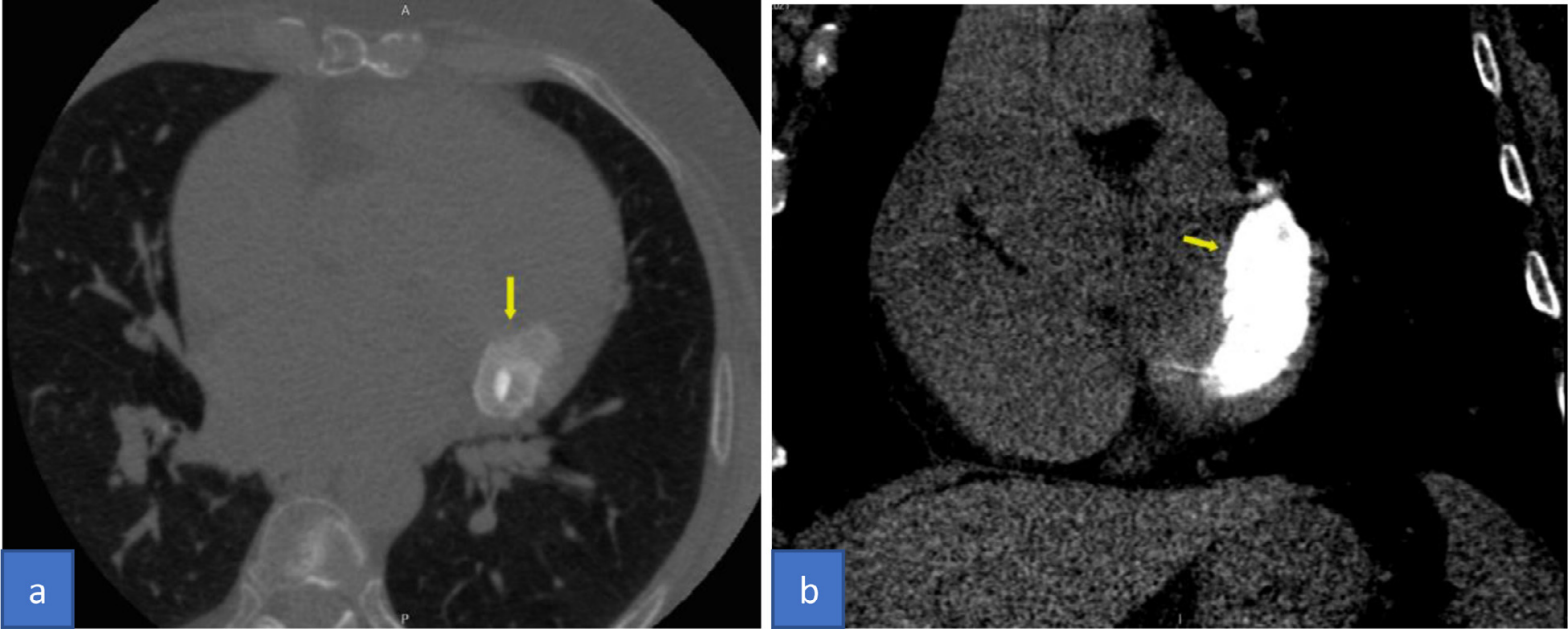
Computed tomography (CT) chest without contrast Axial view lung window (A) and coronal view soft tissue window (B) shows diffuse calcification of the mitral annulus mimicking a hyperdense mass (arrow).
